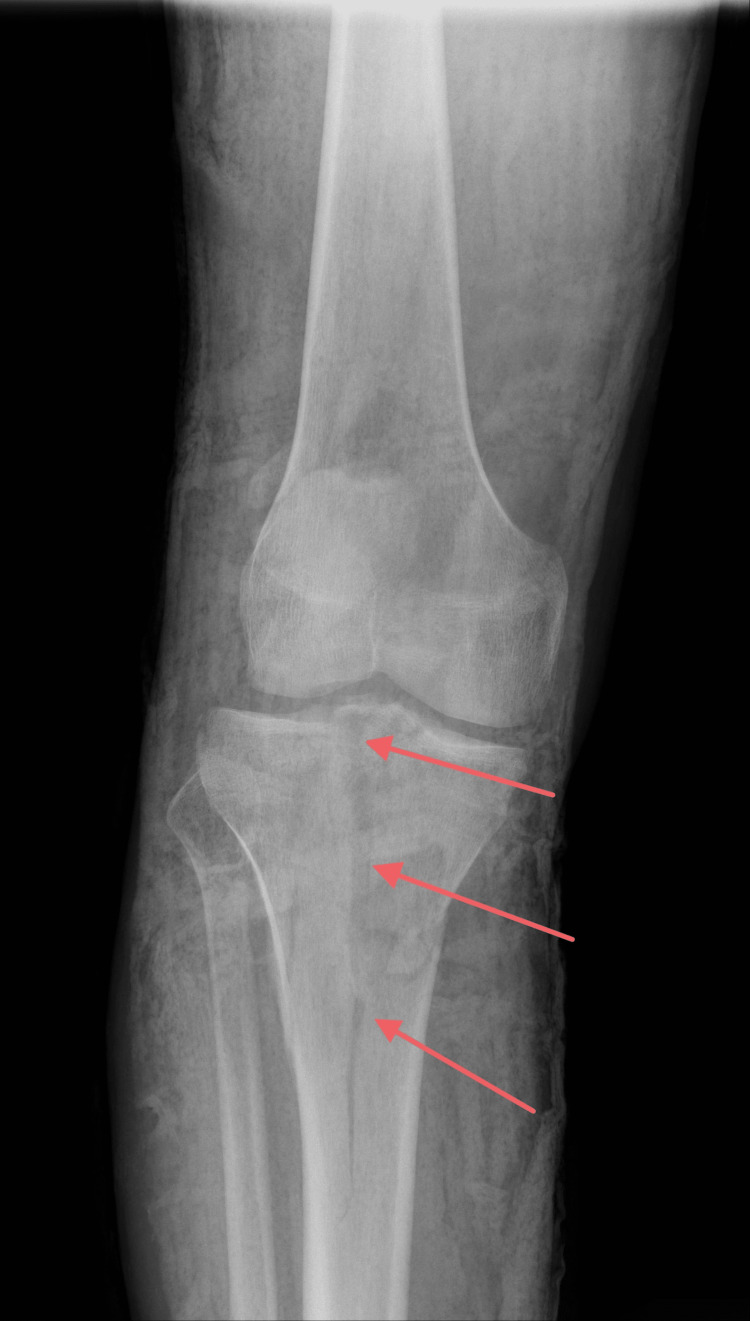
X-ray (AP view) showing a Schatzker type V fracture Red arrows point to the fracture line
